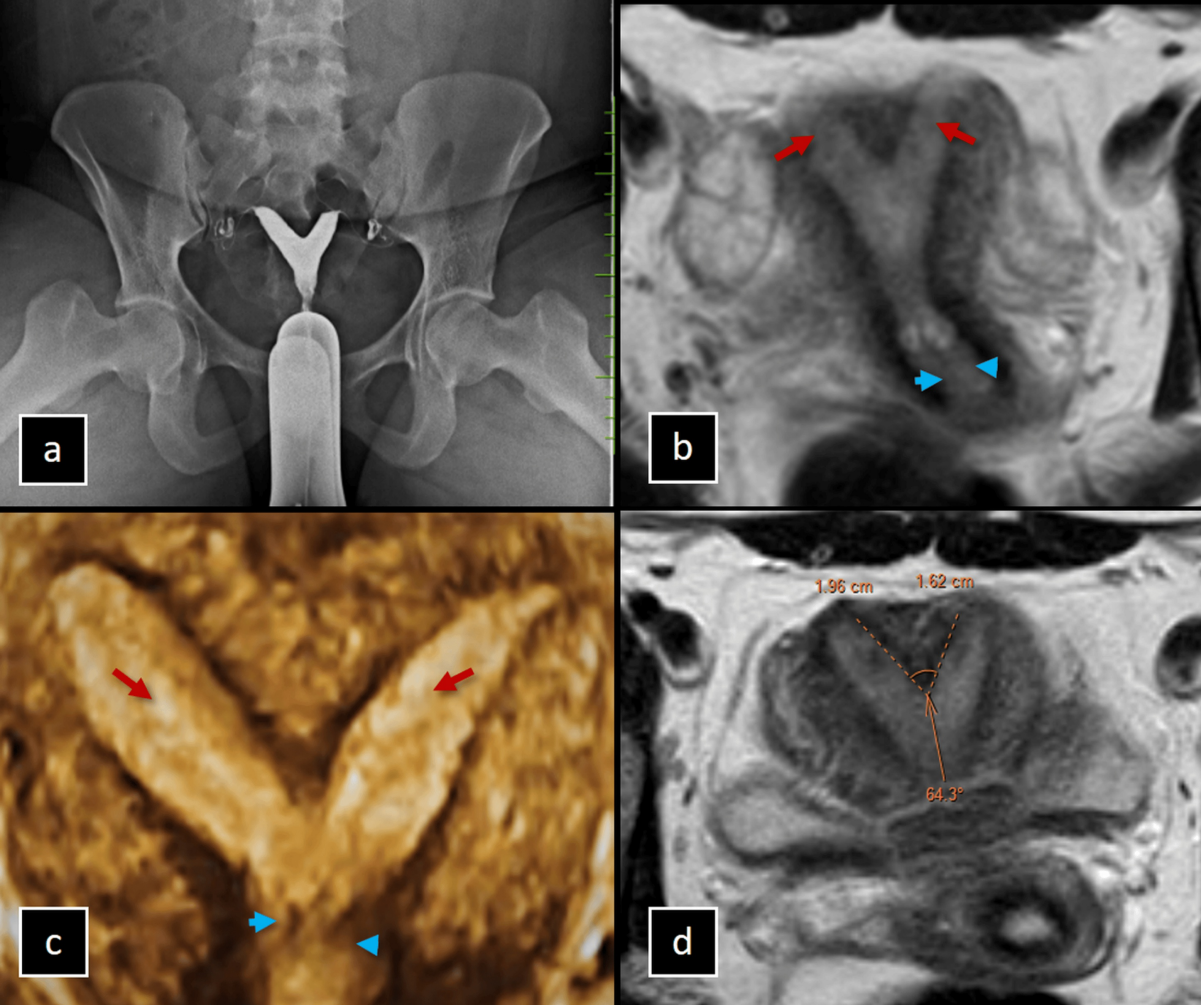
(a-d) Imaging of the Partial Septate Uterus in a Patient With Recurrent Pregnancy Loss. (a) Hysterosalpingogram (HSG) image of a 26-year-old female with recurrent pregnancy loss shows two endometrial cavities at the fundus level, joining above the internal os to form a single cervical canal, suggestive of a septate or bicornuate uterus. (b) MRI T2 oblique axial image demonstrates the two endometrial cavities (red arrows) joining above the internal os to form a single cervical canal (blue arrowheads). (c) 3D ultrasonogram demonstrates the two endometrial cavities (red arrows) joining above the internal os to form a single cervical canal (blue arrowheads). The external uterine contour appears mildly concave with an angle of 64 degrees between the horns. (d) MRI T2 oblique axial image demonstrates partial septate uterus.
